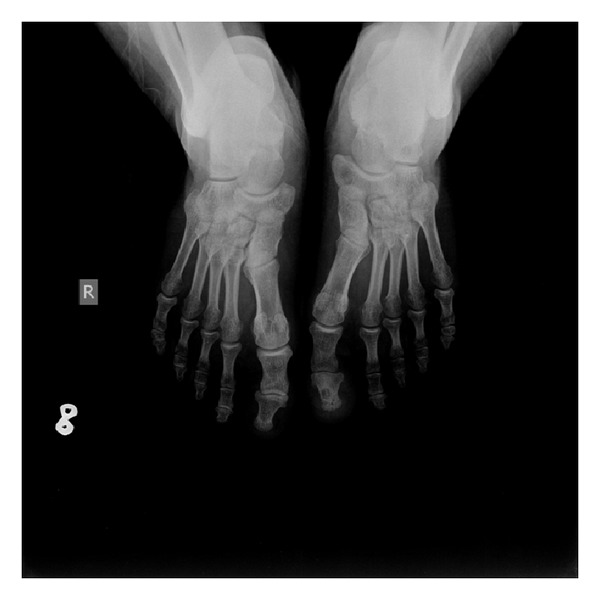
Preoperative AP X-ray of both feet.
